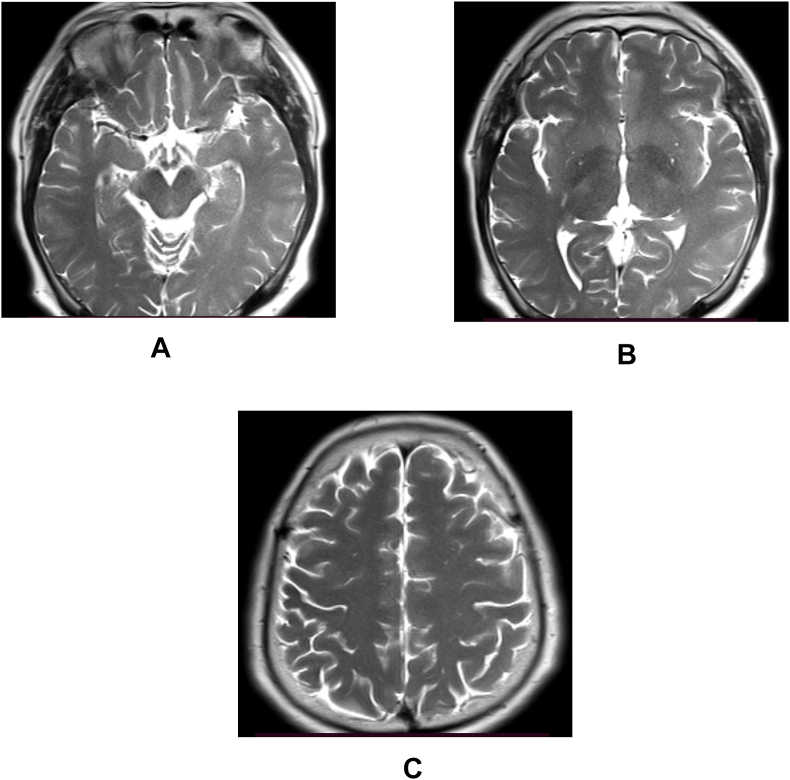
Axial T2-weighted MRI scan Legend (T2_a, T2_b and T2_c): Axial T2-weighted MRI showing normal scan with no any increased signal in corticospinal tracts.
